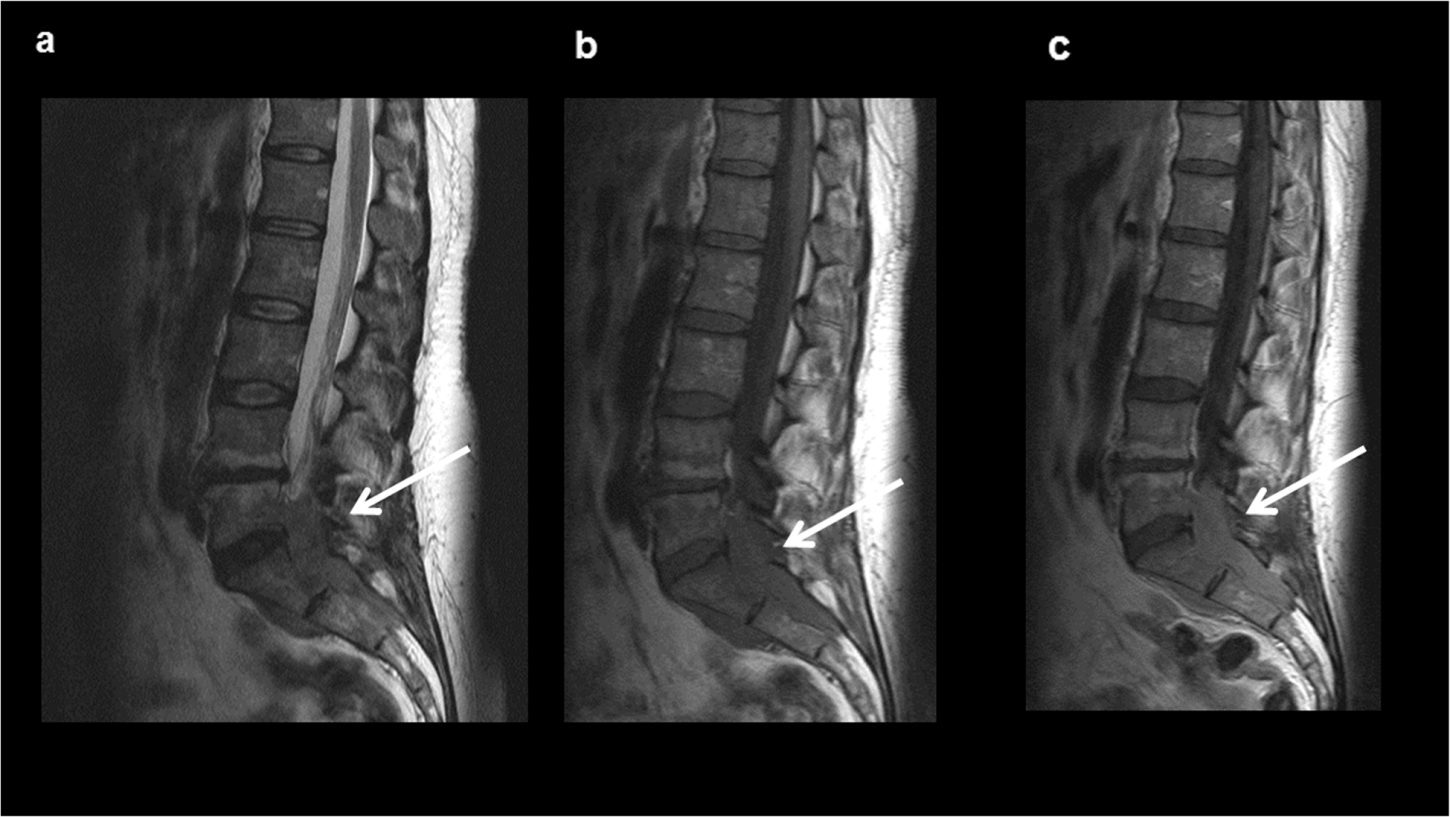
Intraspinal manifestation of Myeloid sarcoma in the lumbosacral region in a female patient with known acute myeloid leukemia in a relapse setting. a On sagittal T2-weighted image, the lesion is homogenous, hyperintense compared to adjacent muscle tissue (arrow). b On sagittal T1-weighted image, the lesion is hypointense (arrow). c On sagittal T1- weighted image after application of contrast medium a strong homogenous contrast enhancement can be appreciated (arrow)
